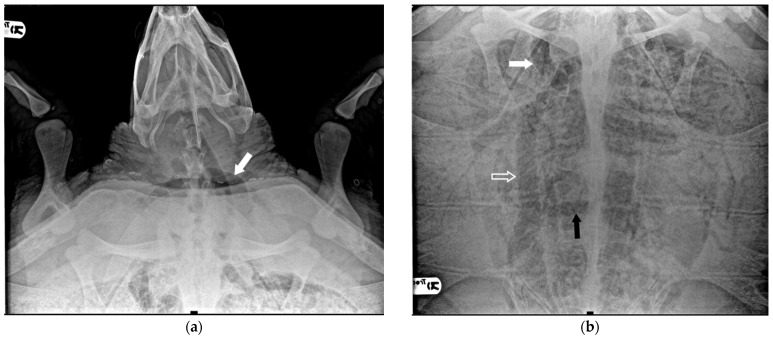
X-ray examination in DV projection of a subject diagnosed with severe GE. An evident massive presence of gas in the majority of vessels: (a) the white arrow shows the subclavian artery; (b) the white arrow shows the massive presence in the precaval vein, the white empty arrow the postcava vein, the black arrow the transverse vein, obscuring a full view of the lung areas.
